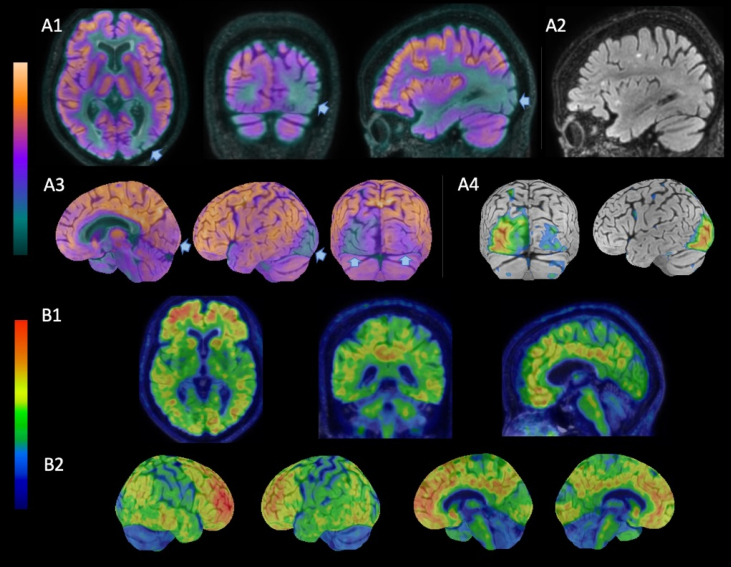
[18F]FDG and [11C] PiB–PET/MRI. (A1) [18F]FDG-PET/MRI: left-predominant bilateral hypometabolism in the occipital and temporo-occipital areas (arrows). (A2) Left sagittal MRI (FLAIR): no structural abnormalities detected. (A3) [18F]FDG-PET 3D-SSP images (Cortex ID suite, GE Healthcare). (A4) Z-score projection of hypometabolism. (B1) [11C]PiB-PET/MRI: diffuse cortical amyloid deposition – transaxial, coronal and sagittal views (B1) and 3D-SSP (B2).
